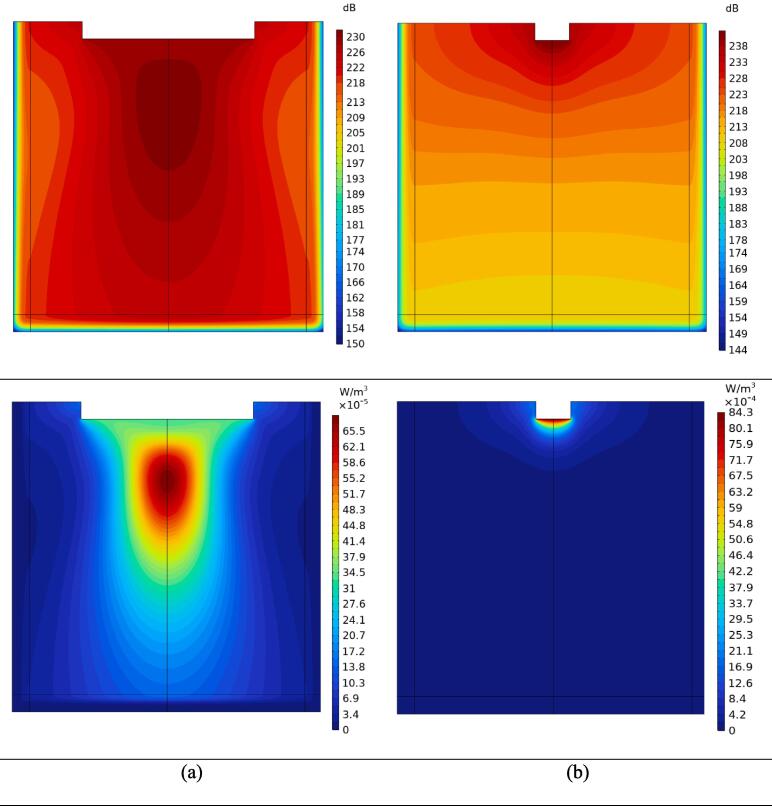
Total ultrasonic pressure (A), total sound pressure level (B), and contours of thermal power dissipation density (C) for probe tip diameter of a) 100 mm and b) 20 mm.
